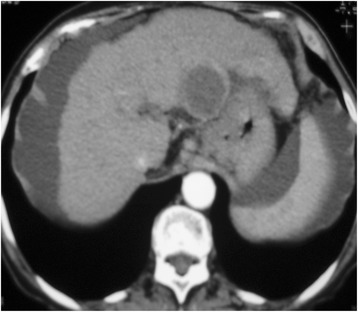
Computed tomography after DEB TACE. Computed tomography 30 days after DEB TACE showing lack of enhancement in the liver tumor consistent with complete response accordingly to the EASL criteria
